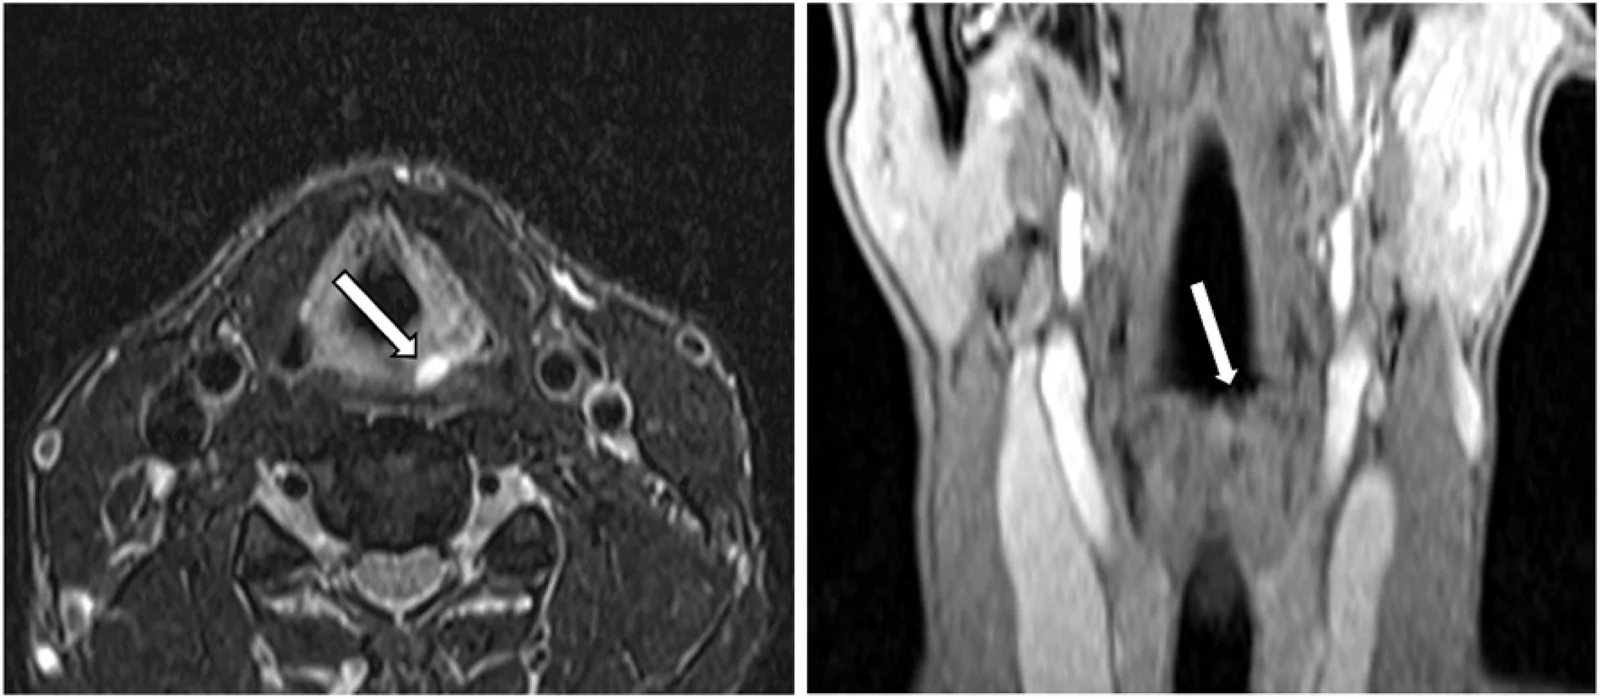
Axial T2-weighted fat-suppressed and coronal post-contrast T1-weighted MR images performed during the second hospitalization demonstrate marked reduction of the inflammatory changes in the aryepiglottic folds, with persistence of a small cystic lesion along the left aryepiglottic fold, without associated inflammatory signs (arrows).
